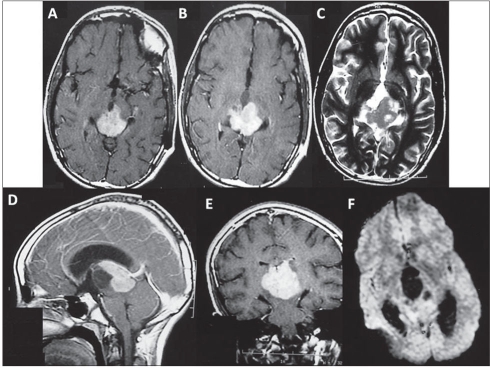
Patient 1: A and B: Contrast enhanced axial T1-weighted MRI following the ventriculoperitoneal shunt revision showed the uniformly enhancing infiltrative lesion occupying the posterior third ventricular region and the quadrigeminal cistern. C: T2-weighted axial image showing the lesion to be heterogeneously iso- to hyperintense. D: Sagittal contrast enhanced T1 image showing the lesion occupying the third ventricle along the internal cerebral vein almost reaching upto the foramen of Monro. E: The coronal-enhanced T1 image showing the vertical extent of the lesion from the foramen of Monro to the midbrain. F: The diffusion-weighted image showing restriction of diffusion within the lesion.
